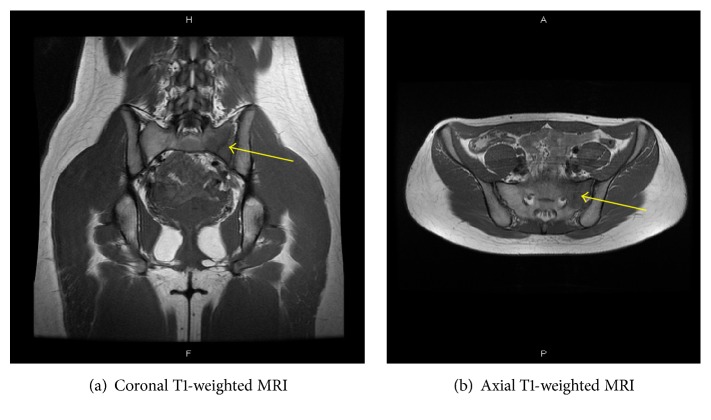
T1-weighted MRI scan shows a linear area of decreased signal intensity of the left sacrum with surrounding a T1 hypointense area (arrow).
